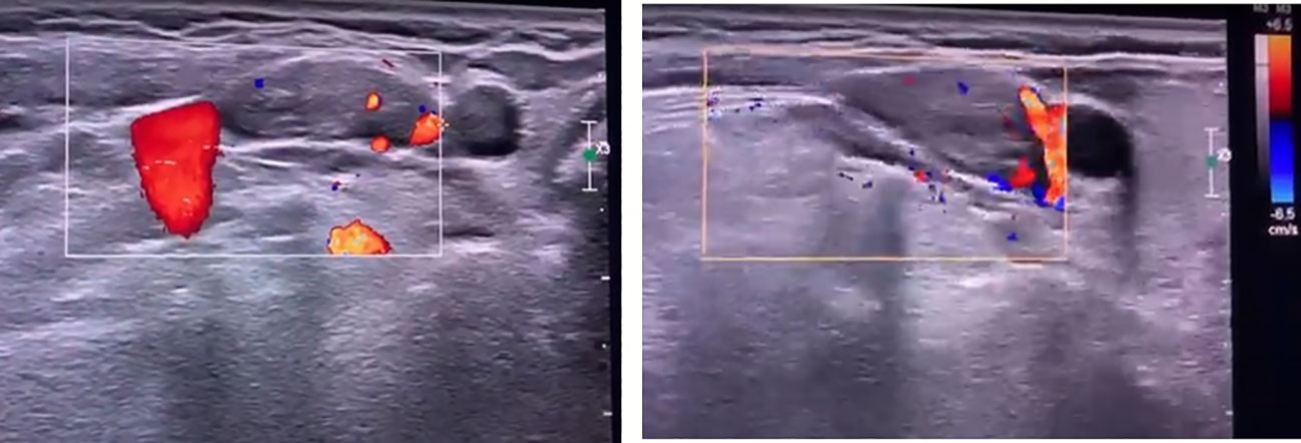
Ultrasound images showing a hypoechoic mass, medial to the internal jugular vein and posteriolateral to the carotid artery.
